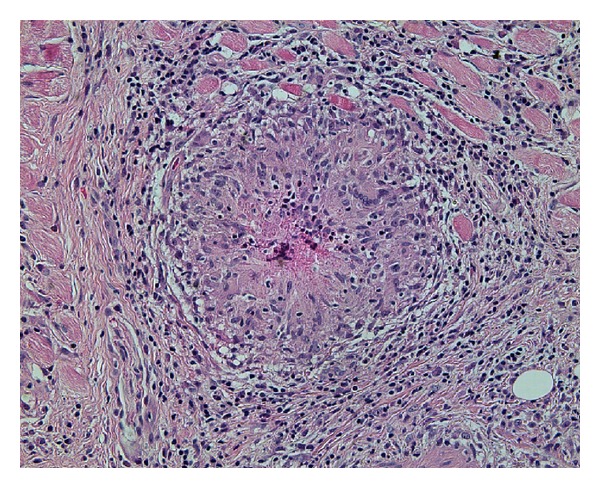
Higher magnification of 20x view showing multinucleated giant cells within the muscle and focal endomysial infiltrates of lymphocytes and plasma cells, consistent with a diagnosis of *orbital sarcoid*, although CT chest and ongoing followup have excluded systemic sarcoidosis.
